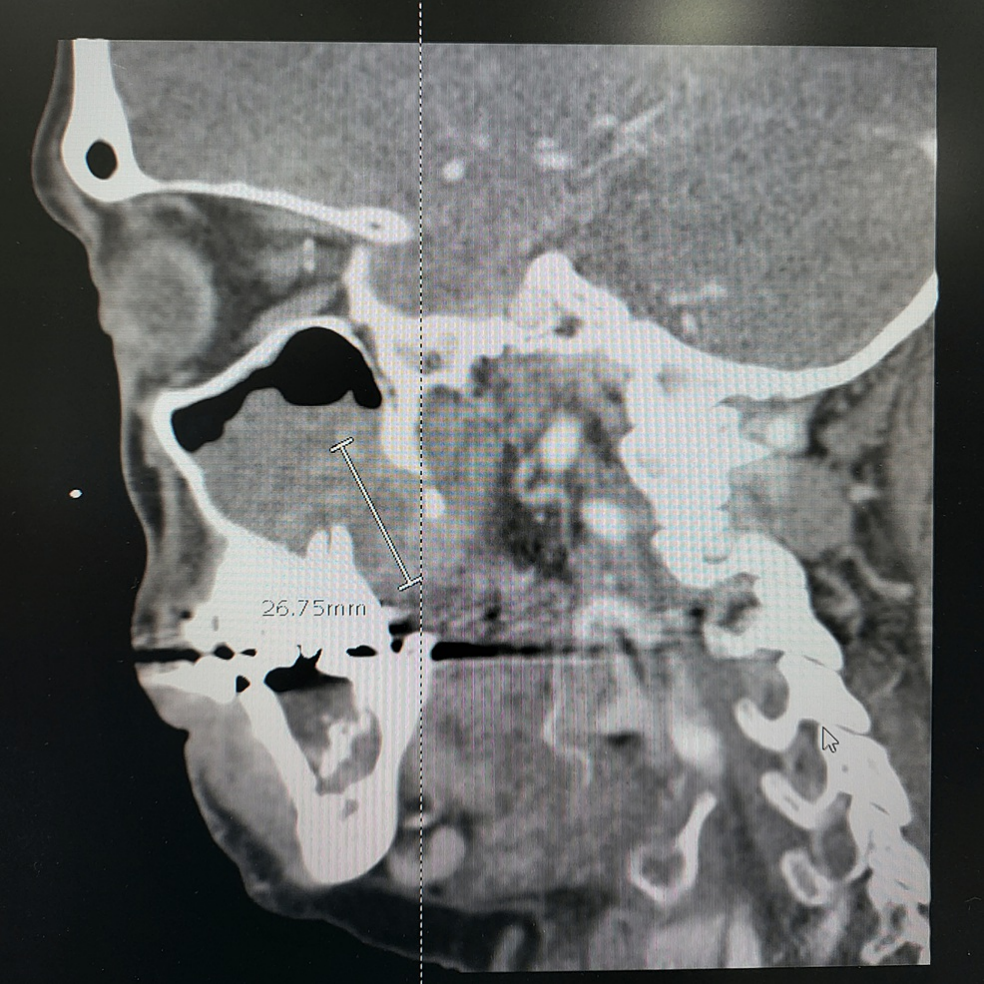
Sagittal CT shows the extent of the lesion. The lesion in this view extends to the pterygoid plates posteriorly.
